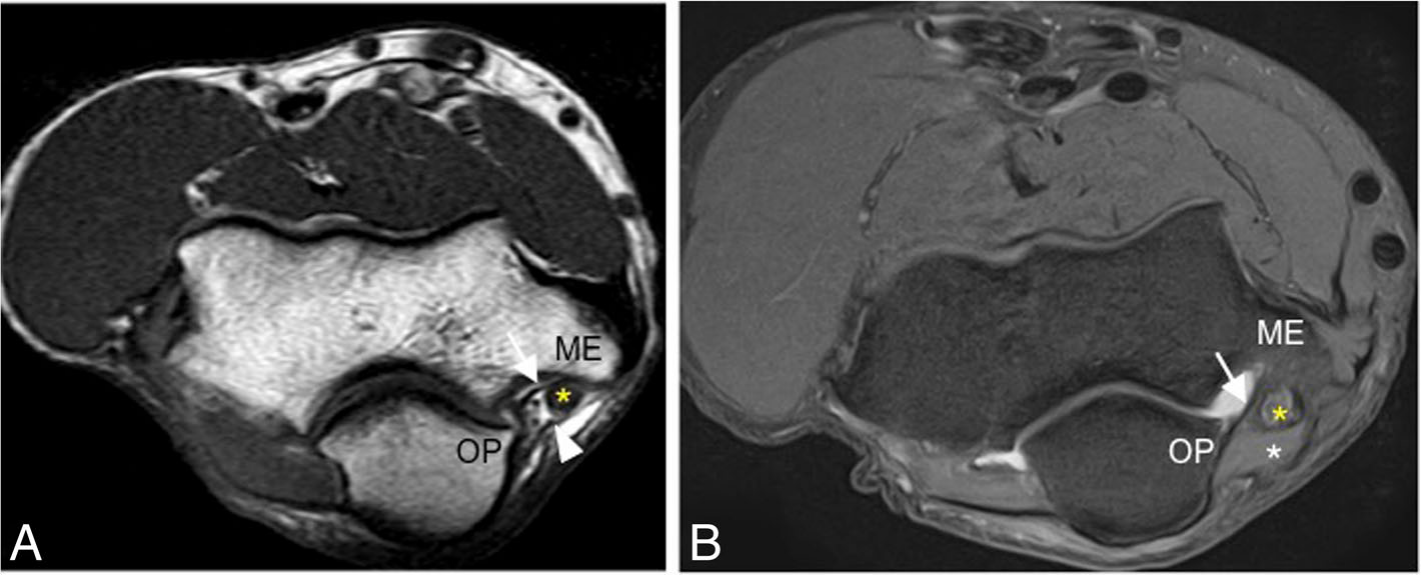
Axial T1-weighted MRI (a) and axial FS PD-weighted MRI (b) in two different healthy volunteers showing the intact posterior bundle of the medial collateral ligament complex (white arrows). It extends from the posterior aspect of the medial epicondyle (ME) to the medial aspect of the olecranon process (OP). Cubital tunnel retinaculum (white arrowhead). Ulnar nerve (yellow asterisks). Anconeus epitrochlearis muscle (white asterisk)
